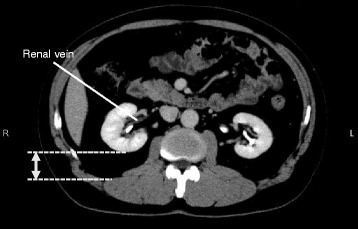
The methodology of measurement of retroperitoneal fat tissue thickness using axial computed tomography imaging. The measurement of retroperitoneal fat tissue thickness is performed at the slice that showed the renal vein on the treated kidney side
